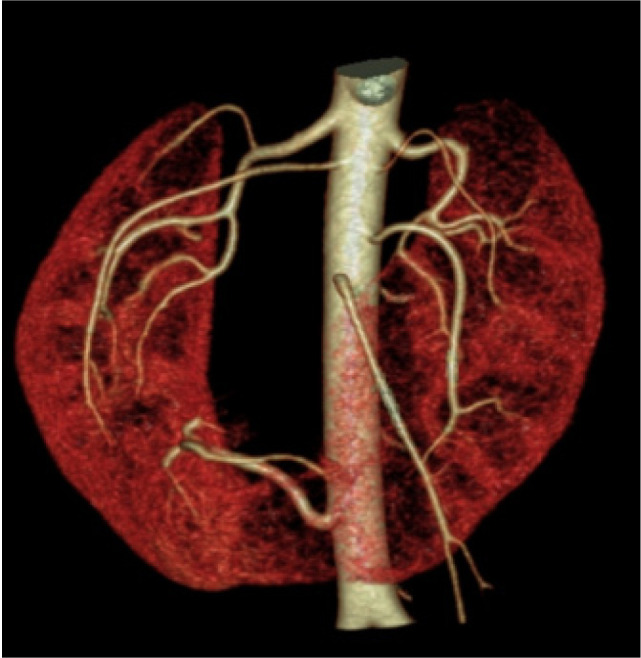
Three-dimensional volume rendering CT angiography showing renal and accessory arteries on either sides supplying both kidneys. Another artery arising from infra renal aorta supplying isthmus, tumor, and adjoining lower pole of right kidney.
